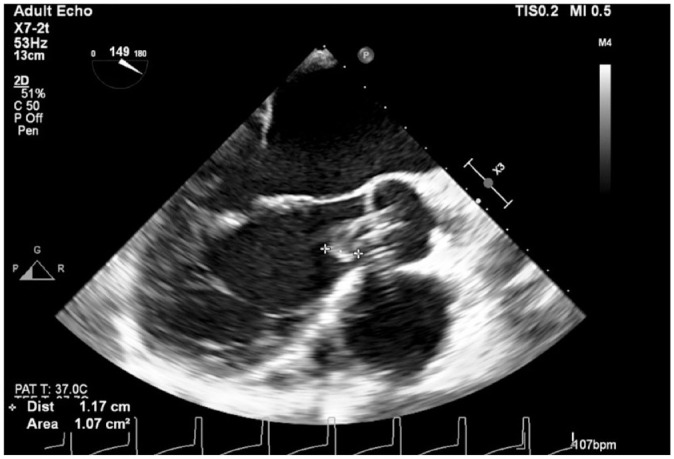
Transesophageal echocardiogram (long-axis aortic view) showing the Impella cannula (Abiomed, Danvers, MA, USA) in the left ventricle with a 1 cm clot on the cannula toward the inflow portion.
